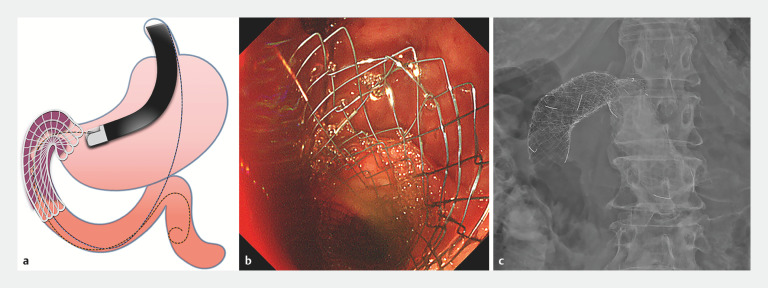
Successful placement of a gastroduodenal stent using the rendezvous technique with endoscopic ultrasound is shown on:
